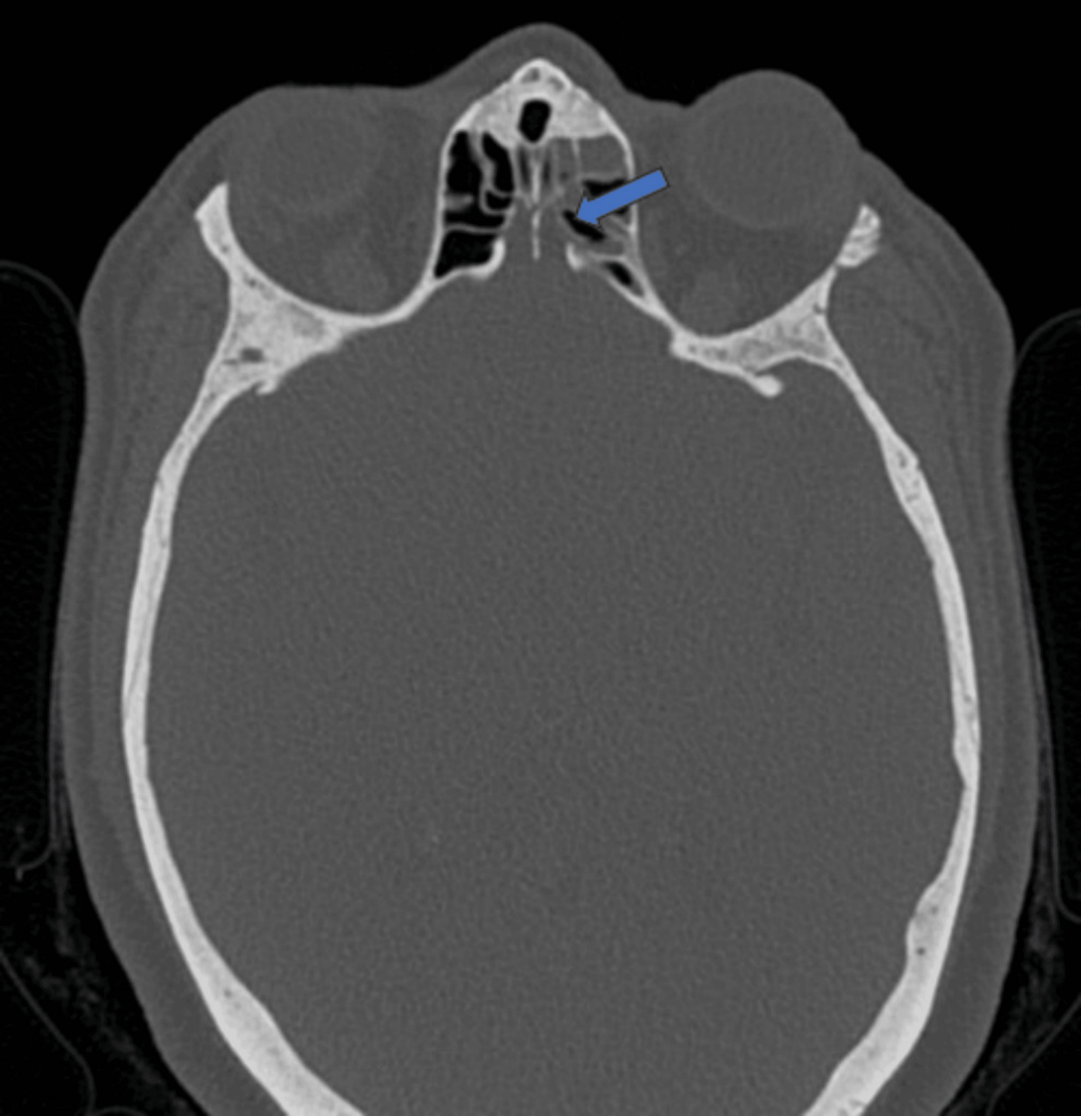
Axial section of CT paranasal sinus at the level of cribriform plate The image shows a focal dehiscence (blue arrow) in the left cribriform plate.
